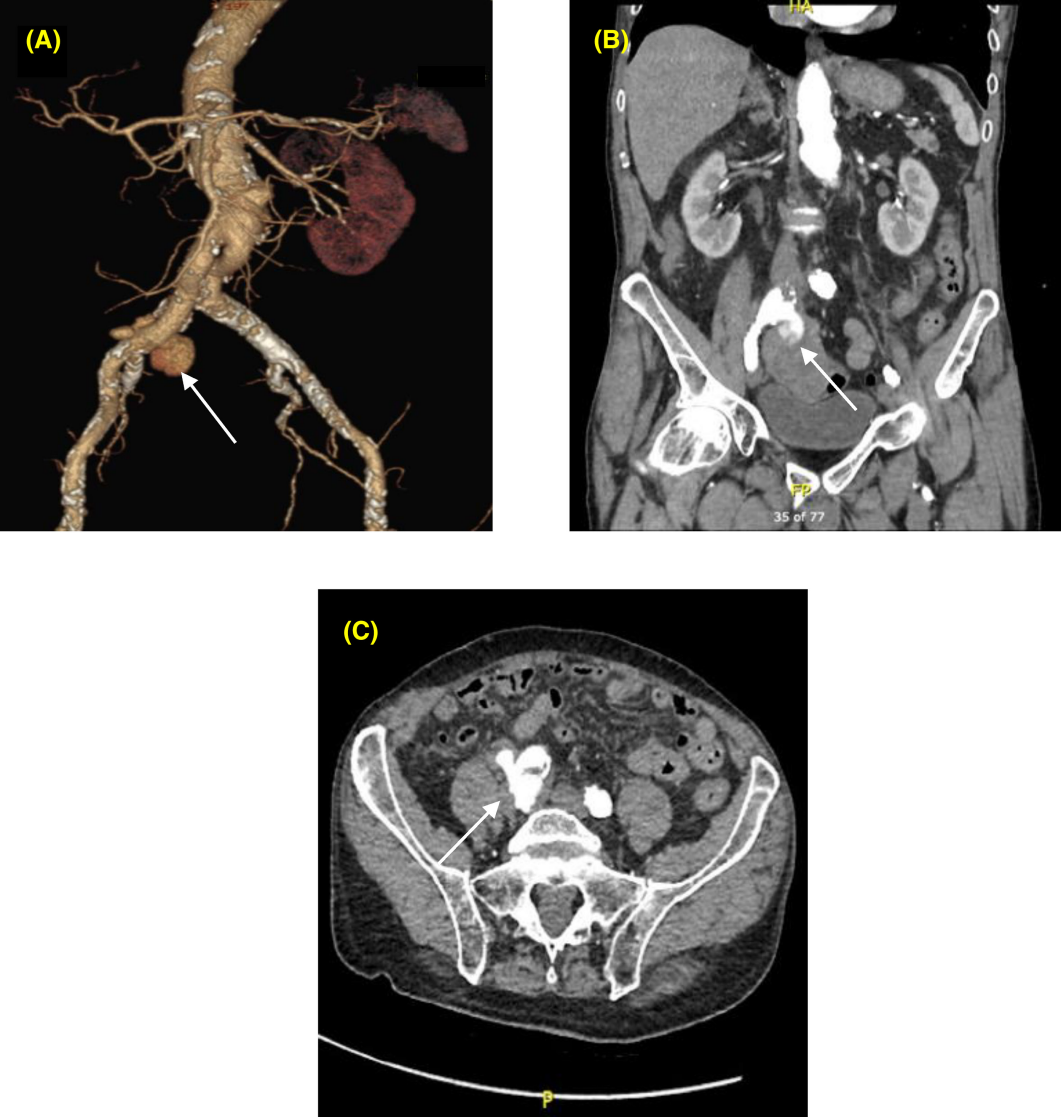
Computed tomography (CT) findings. (A) Digital subtraction CT angiography image demonstrating right common iliac artery anastomotic pseudoaneurysm (white arrow). (B) Coronal CT image demonstrating the site of fistulation into the sigmoid colon. (C) Axial image demonstrating the anatomy of the pseudoaneurysm.
